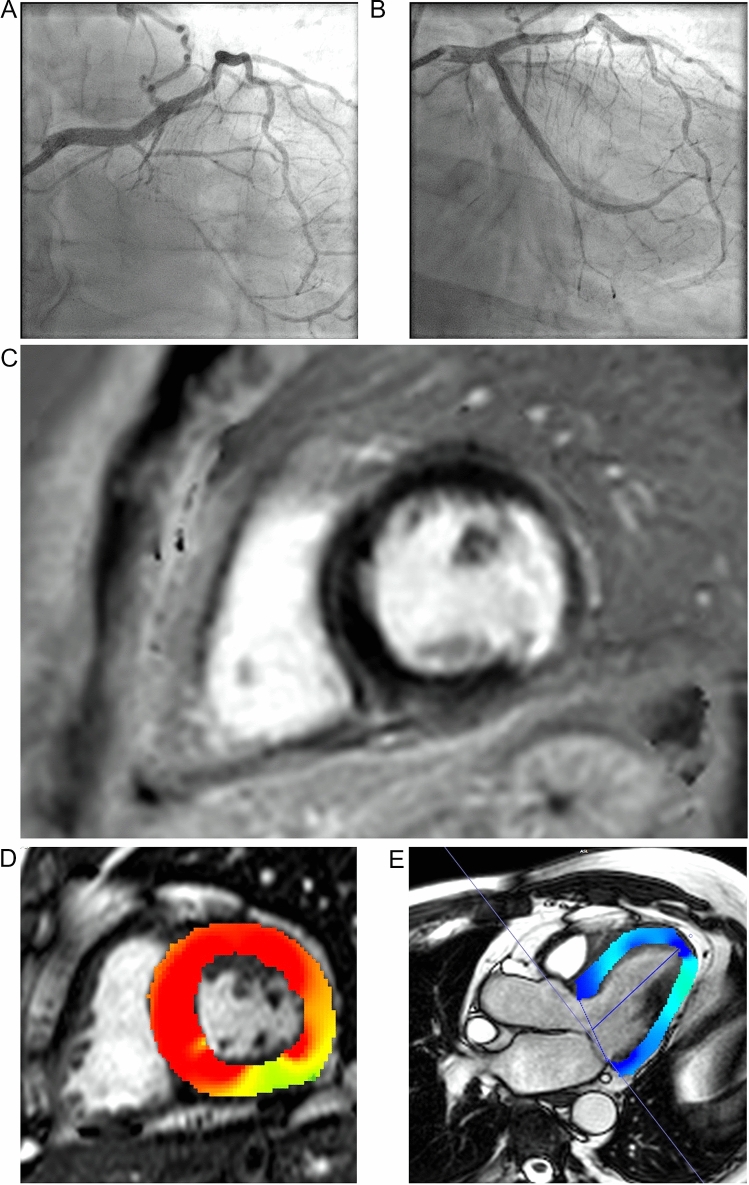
Example of a patient with chronic total occlusion of the left circumflex (LCX). A Angiography before and B after percutaneous coronary intervention. The cardiac magnetic resonance imaging prior to the revascularisation demonstrated C non-transmural scarring in the late-gadolinium enhancement (LGE) image and D hypokinesia of the lateral or posterolateral wall, as seen in the colour-encoded images of the radial strain D and E longitudinal strain
